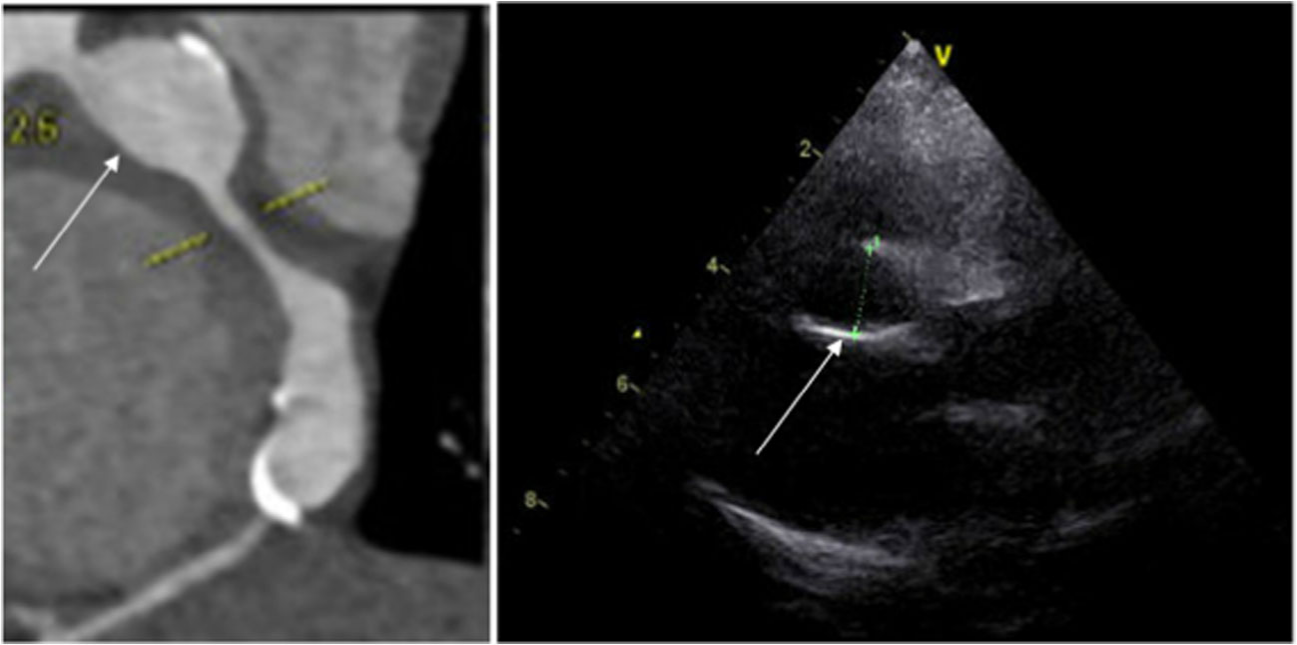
Proximal and distal aneurysm in the RCA with calcification depicted by cCTA vs echocardiography
